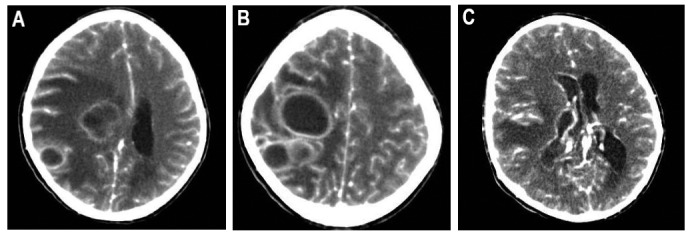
Computed tomography brain image showing rim-enhancing collections in the right frontal (A) and parietal lobe with mass effect (B).
